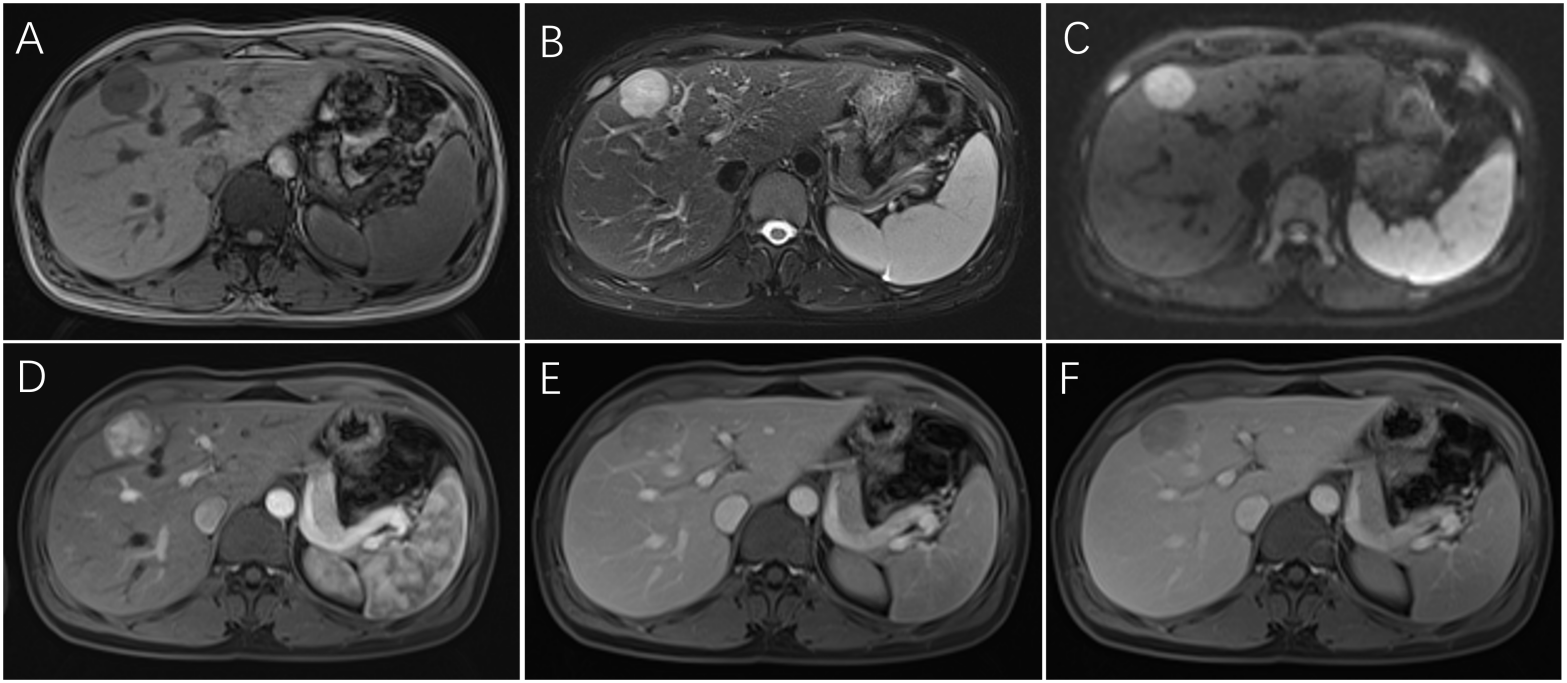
Dynamic MRI of hepatic PEComas. A 33-year-old woman with hepatic PEComa was admitted for the presence of liver mass with non-specific symptoms, who was first misdiagnosed as hepatocellular carcinoma on imagings. MRI showed a regular well-defined mass in segment IV of the liver, with hypointensity on T1-weighted images (A), hyperintensity on fat-suppressed T2-weighted images (B), and hyperintensity on diffusion-weighted images (C). The tumor was significantly heterogeneous enhanced and showed high signal in the arterial phase (D), which decreased rapidly in the portal venous phase with fast-washout pattern (E) and had a lower signal in the delayed phase in comparison with the surrounding hepatic parenchyma (F). (PEComa, perivascular epithelioid cell tumor; MRI, magnetic resonance imaging).
